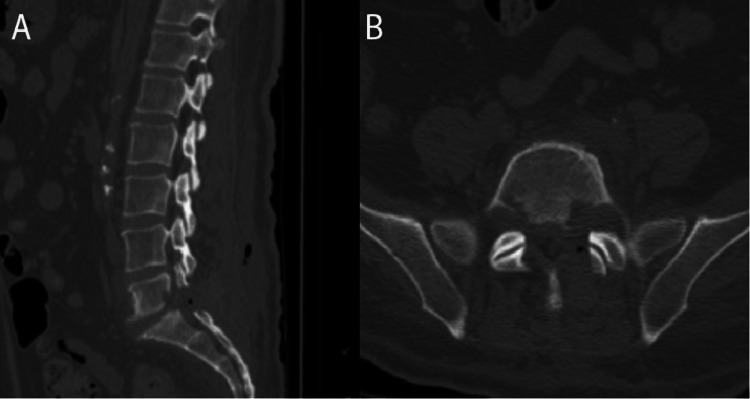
Postoperative CT lumbar spine in A. Sagittal view and B. Axial view, exhibiting interval removal of L5-S1 lamina, medial facet, calcified disc herniation, and part of the L5 vertebral body
